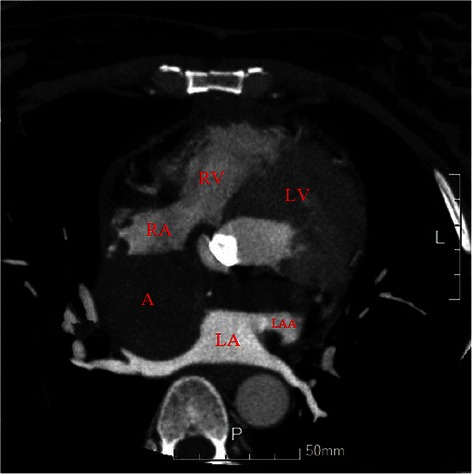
Postoperative CTA coronary angiography showed the thrombosis of aneurysm and fistulous connection. Abbreviations LV: Left ventricle; LA: Left Atrium; RV: Right ventricle; RA: Right Atrium; A: Aneurysm; LAA: Left Atrium Appendix
